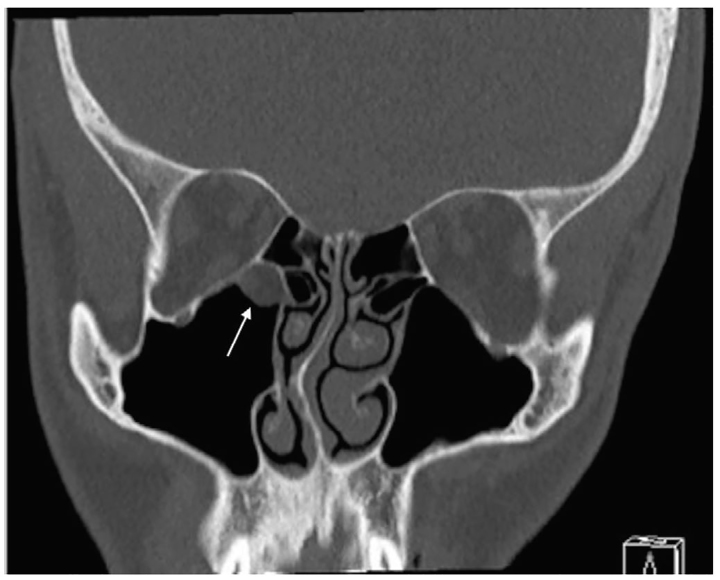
Coronal non-enhanced computed tomography in bone window: Soft tissue density noted in the maxillary antrum and no definite fracture. Lesion initially called a mucosal retention cyst (arrow).
